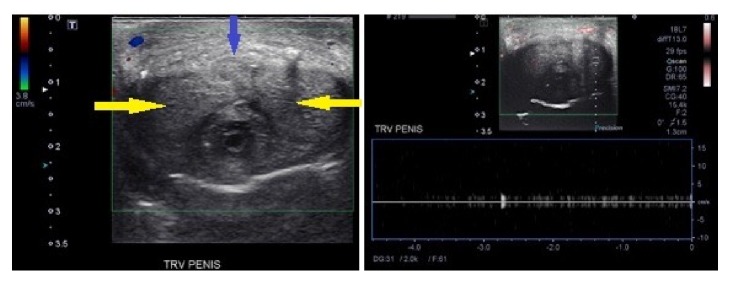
Penile ultrasound Doppler showing no demonstrable arterial flow within the heavily calcified penile arteries (yellow: cavernosal; blue: dorsal).
